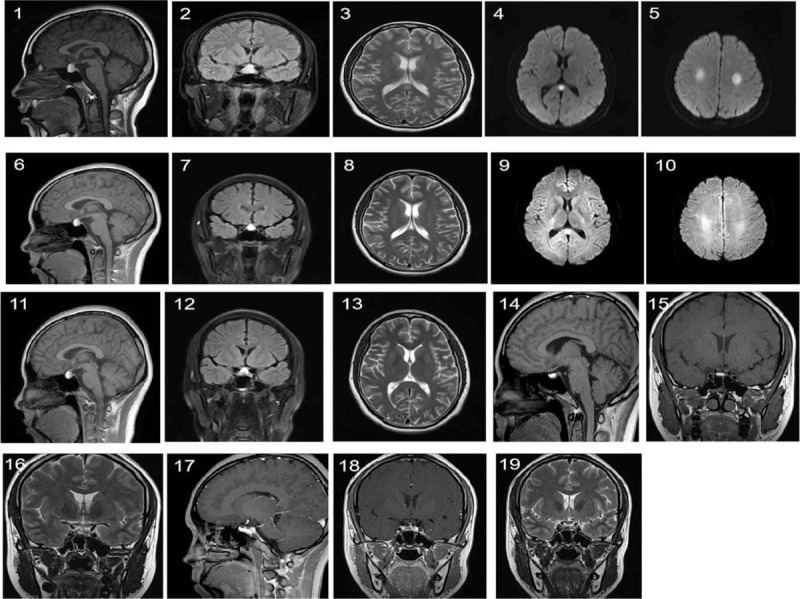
Brain Magnetic resonance imaging of the patient. (A) sagittal T1-weighted images shows a sellar and suprasellar high signal with the size of 15mm × 14mm × 14 mm, in which stratification was demonstrated, (B) coronal FLAIR images shows the high signal appeared as an hourglass, (C) axial T2-weighted images shows hyperintensity in bilateral basal ganglia region and corpus callosum, (D) (E) axial DWI images shows hyperintensity in corpus callosum and bilateral centrum semiovale (picture A–E, during 32 + 1 gestational week on December 12th,2018), (F) (G) shows the size of sellar and suprasellar high signal is reduced to 13mm × 12mm × 13 mm, compared with the previous examination (picture 1, 2),(H) shows the lesion in corpus callosum was slightly smaller, compared with picture 3,(I) (J) the signal of the lesion in corpus callosum and bilateral centrum semiovale was weaker, compared with picture 4, 5 (picture F–J, on December 20th,2018), (K)(L)shows the size of sellar and suprasellar high signal was reduced to 10mm × 11mm × 12 mm, (M)shows the abnormally high signal in bilateral basal ganglia region basically disappeared (picture K–M, on January 18th,2019), (N)(O)(P)shows the size of sellar and suprasellar high signal was further reduced to 8mm × 5mm × 9mm (on April 27th,2019),(Q)(R)contrast enhanced images shows the pituitary gland was almost normal in size, with homogeneous enhancement after enhancement, and pituitary stem was slightly shifted to the left, (S) coronal T2-weighed images shows the pituitary gland appeared as heterogeneous signal (on September 24th,2019).
